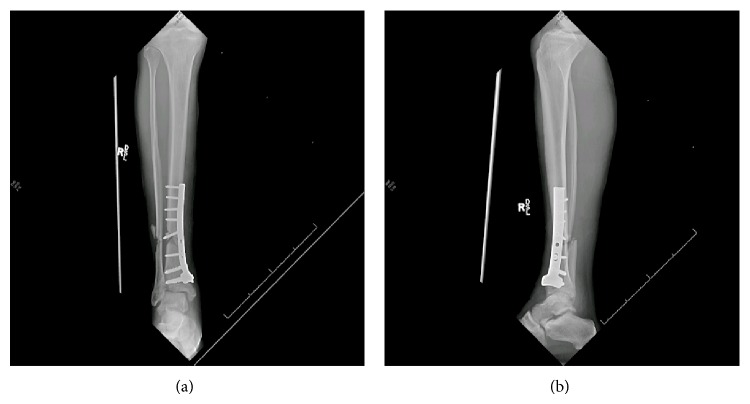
((a) and (b)) Radiographs of an atrophic nonunion of the tibia with hardware failure one year after motorcycle collision resulting in an open tibial fracture. In this patient, little or no callus is evident.
